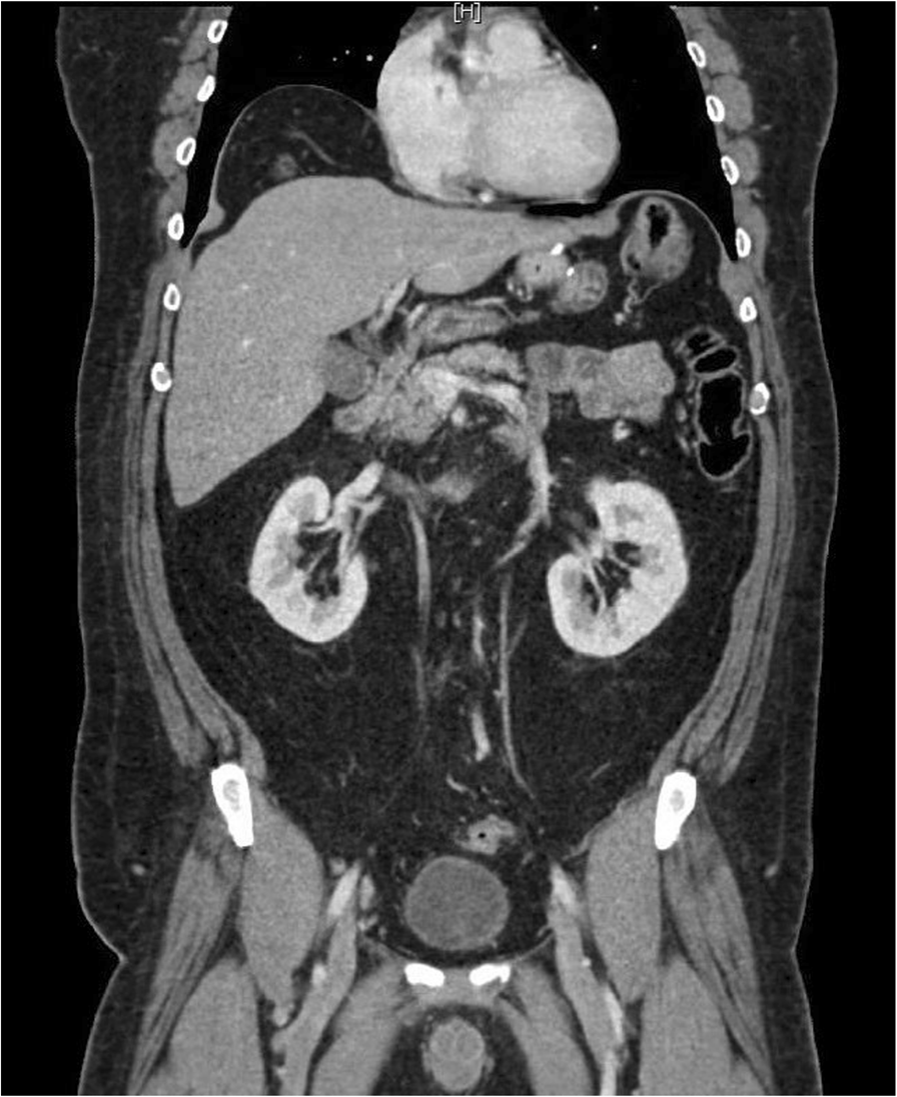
Coronal computed tomography scan of the abdomen and pelvis with contrast showed a loop of colon beneath the right copula of the right diaphragm
